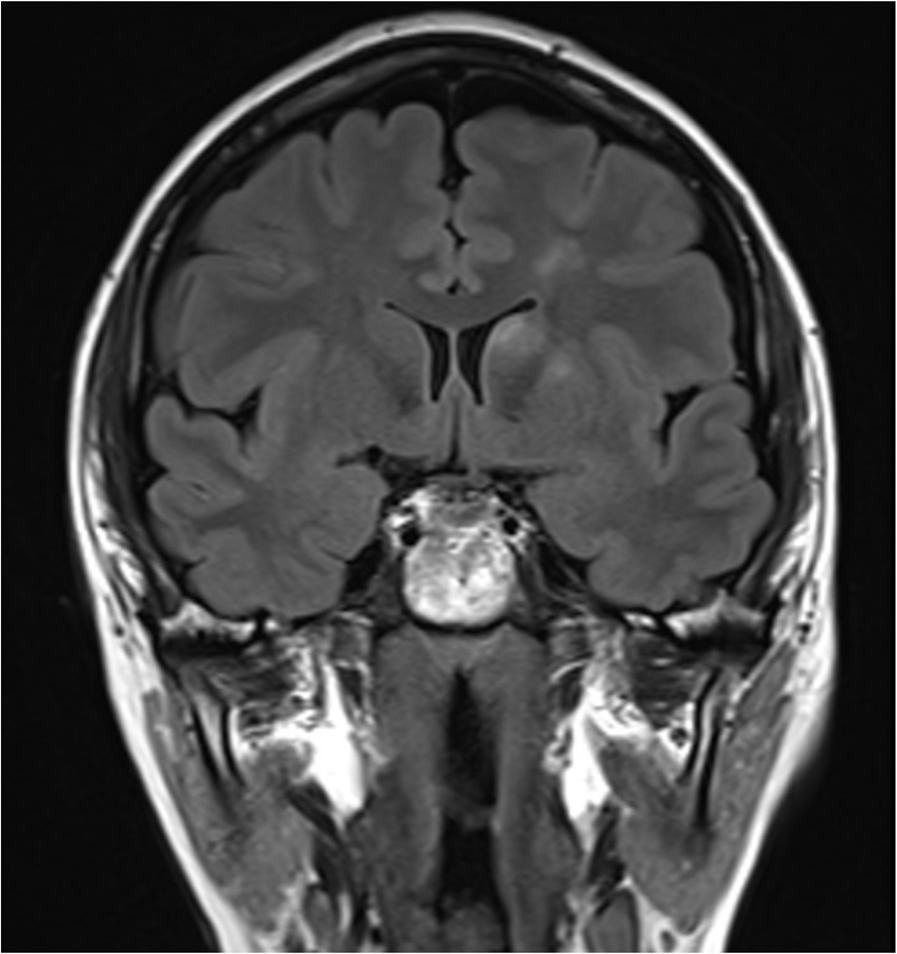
3 Tesla Cerebral MRI coronal FLAIR sequence showing the largest ischemic lesions located in the left cerebral hemisphere
